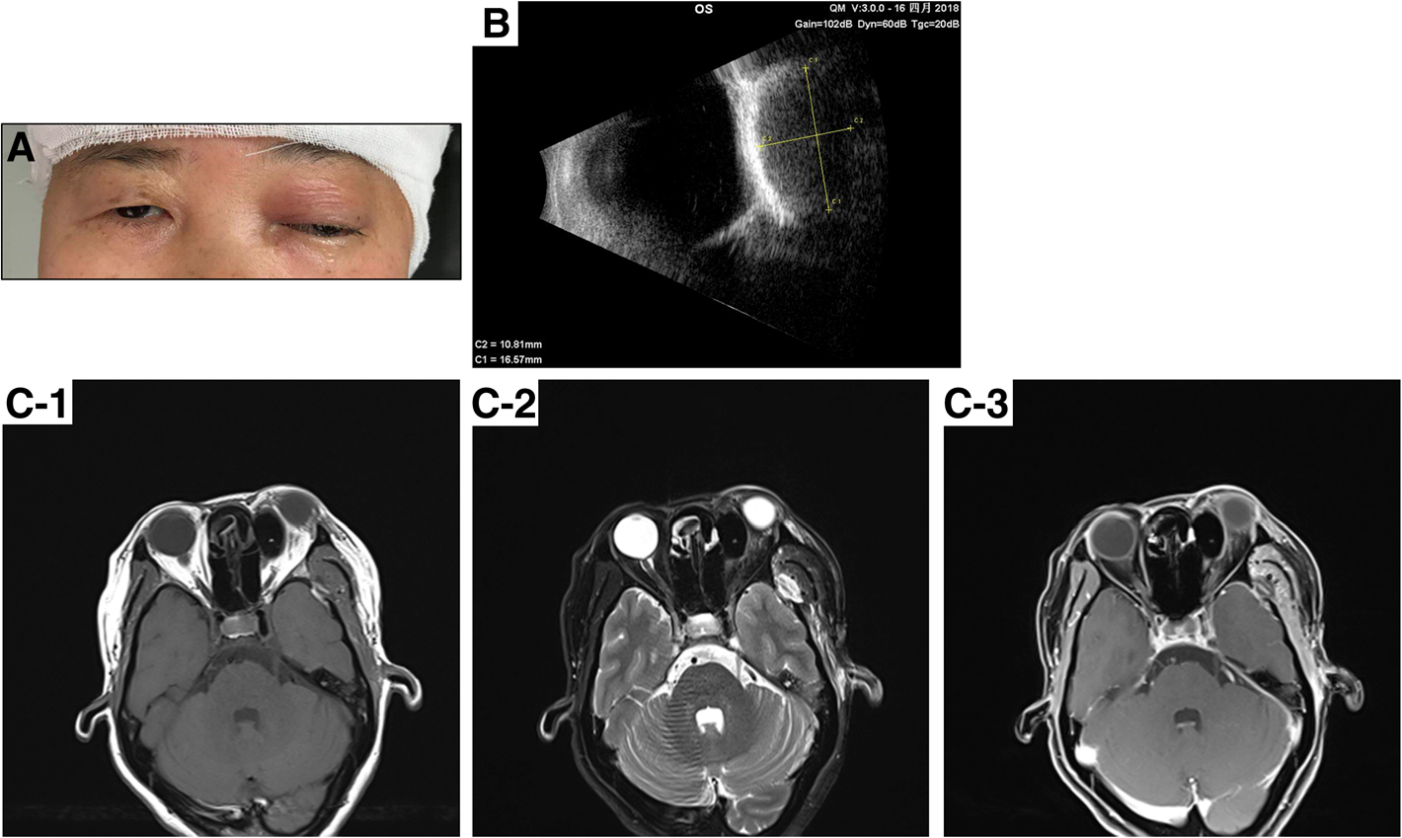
a: The left eye proptosis with ptosis, eyelid swelling; b: B-scan revealed that an oval mass with regular shape in the left orbit, size 10.81 mm X 16.57 mm approximately. c: Orbit MRI revealed that a low T1 and T2 signal mass with regular oval shape was detected in the upper nasal side of left orbit, without enhancement, the left optic nerve, superior rectus muscle and left eye ball were significantly compressed
